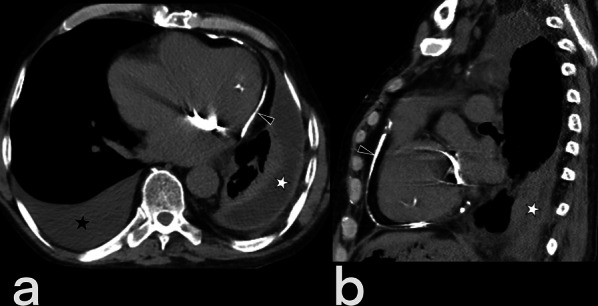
Transverse (a) and sagittal reformatted (b) CT images without intravenous contrast of a 55-year-old male. Pericardial thickening with linear calcification (arrowheads), right-sided pleural effusion (black asterisk), and infected fluid collection (white asterisk) in the left pleural cavity are seen. Mediastinal and pericardial tuberculous
